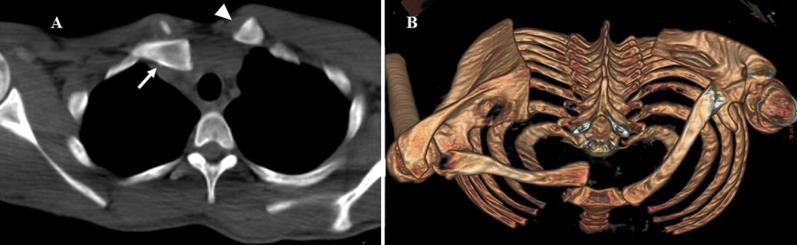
computerized tomography (CT) scan depicting axial (A) views of a right posterior sternoclavicular joint dislocation (arrow) and normal positioned left sternoclavicular joint (arrowhead) and 3D reconstruction (B) (notice the narrowed space behind manubrium on the 3D-reconstruction image)
